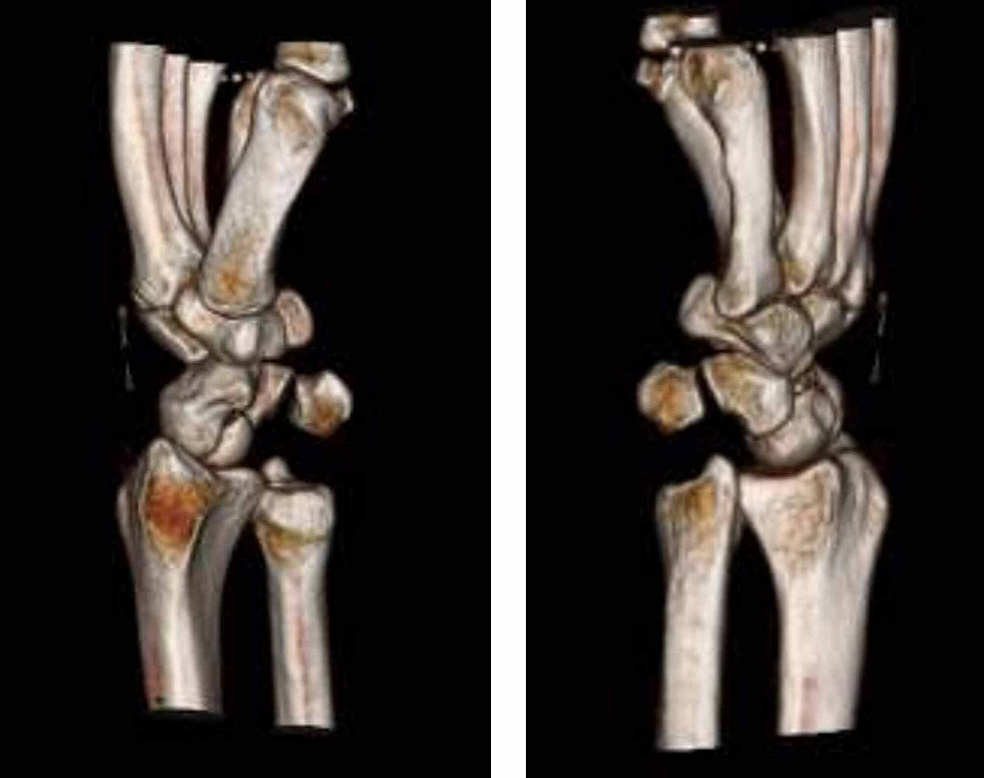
Three-dimensional CT reconstruction of the left wrist CT: computed tomography
